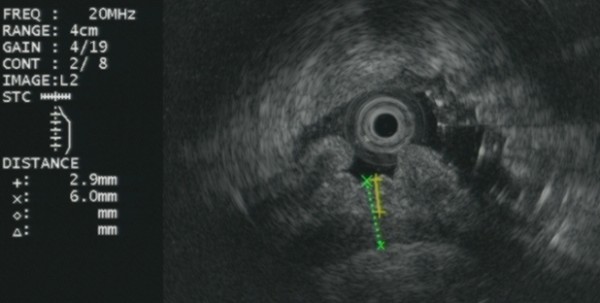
Endoscopic ultrasound shows mild thickening of the mucosa with normal stratification of the gastric wall.
